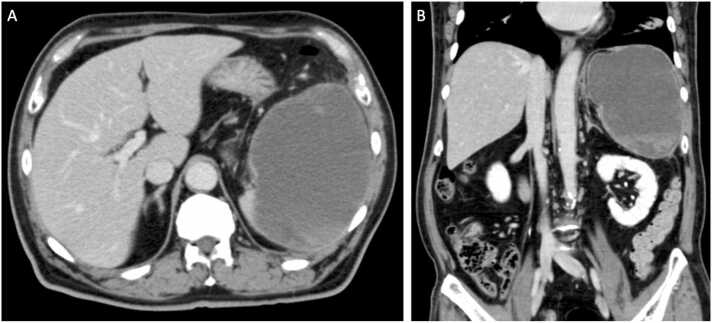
Contrast-enhanced CT of the abdomen on admission. It demonstrates a solitary hypodense lesion measuring 15 × 10 cm. Splenic capsule is thinned around the entire circumference.
